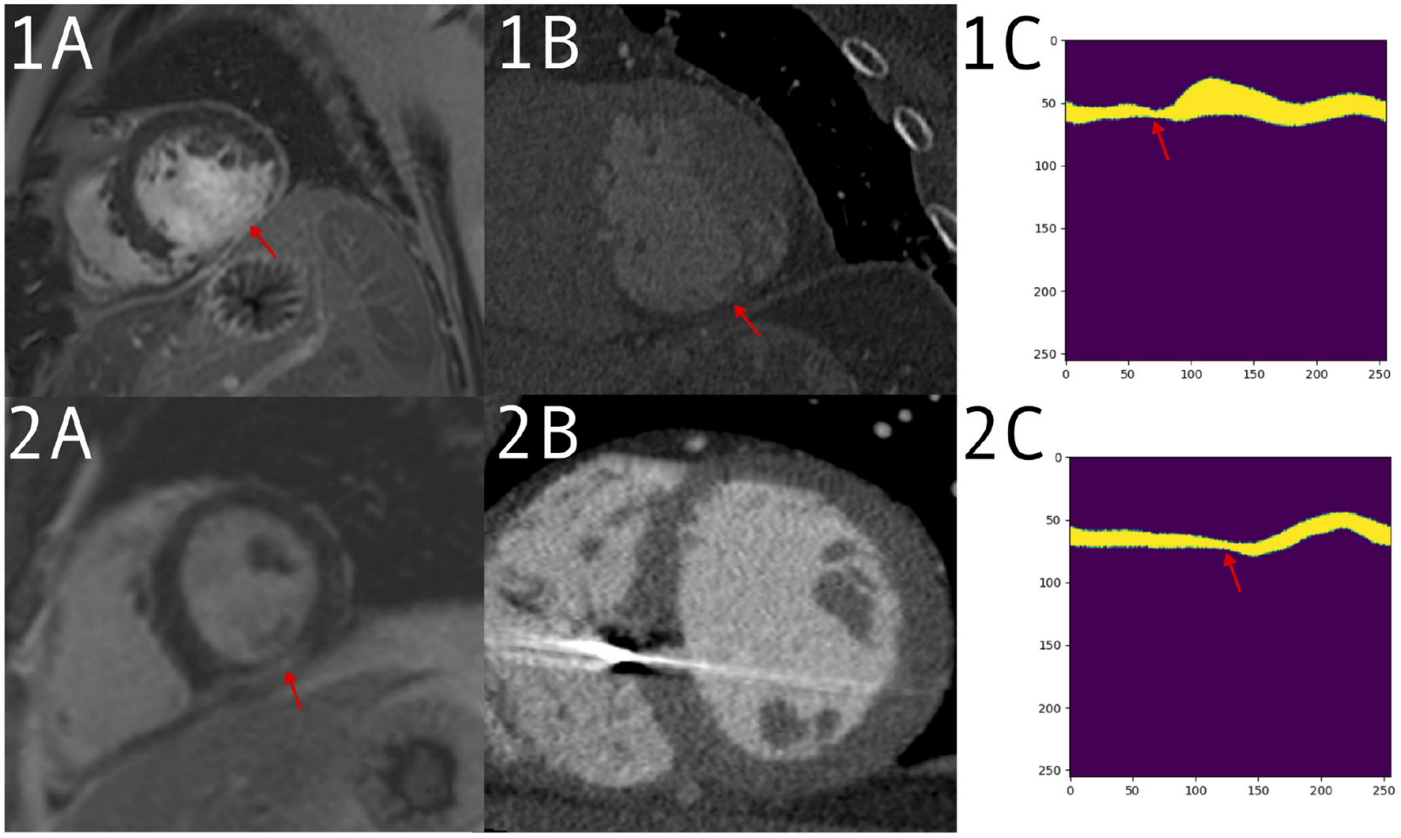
1: Patient with a large defect, detected correctly by both clinician and prediction network. Imaging acquired for valve replacement procedure and decreased left ventricle (LV) function from suspected infarction. (1A) A large defect on the late gadolinium enhancement (LGE) scan. (1B) The computed tomography angiography (CTA) with thinning visible in the same region. (1C) The input to the network for a mid-ventricle slice, in the form of a polar coordinates myocardium mask derived from the CTA segmentation. Thinning can be seen around the red arrow. (2) Patient with a smaller defect and less remodeling, which was detected by the network but not the clinician. Imaging performed for VT ablation planning. (2A) A positive LGE area on a mid short-axis slice. (2B) A CTA slice without an obvious defect visible. (2C) The polar mask with a change in anatomy, which is picked up by prediction network as a possible scar.
